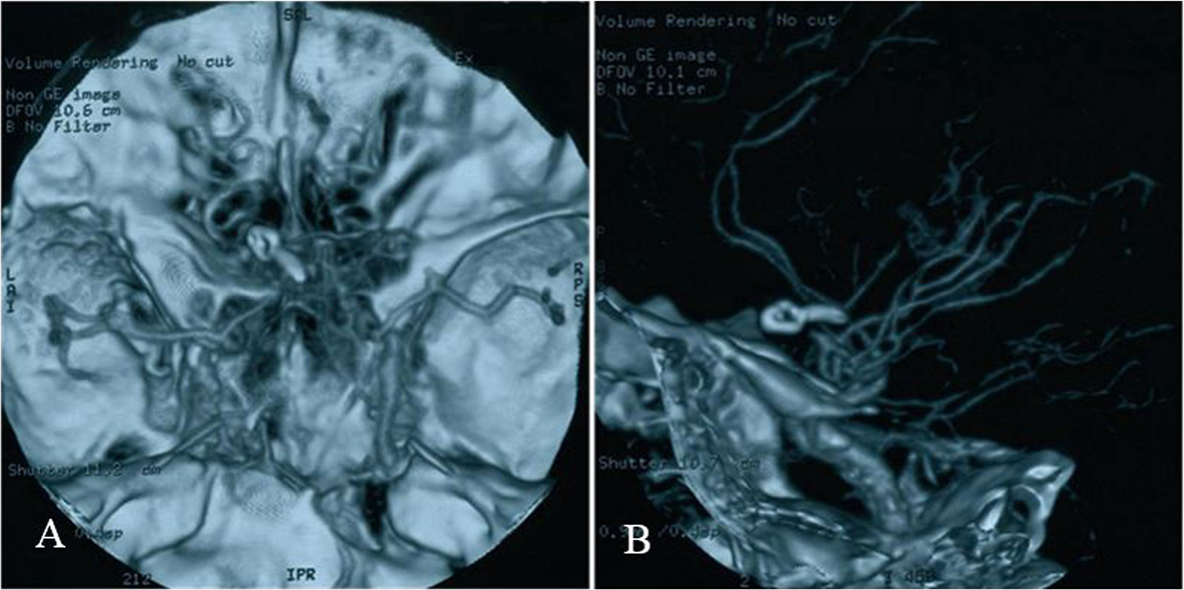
CTA after aneurysm clipping. a, b The image of the aneurysm clip is clearly displayed. The anterior communicating artery aneurysm was completely clipped and closured. There was no residual neck of the aneurysm. The morphology and course of the Willis Circle was normal, without compression and displacement changes
